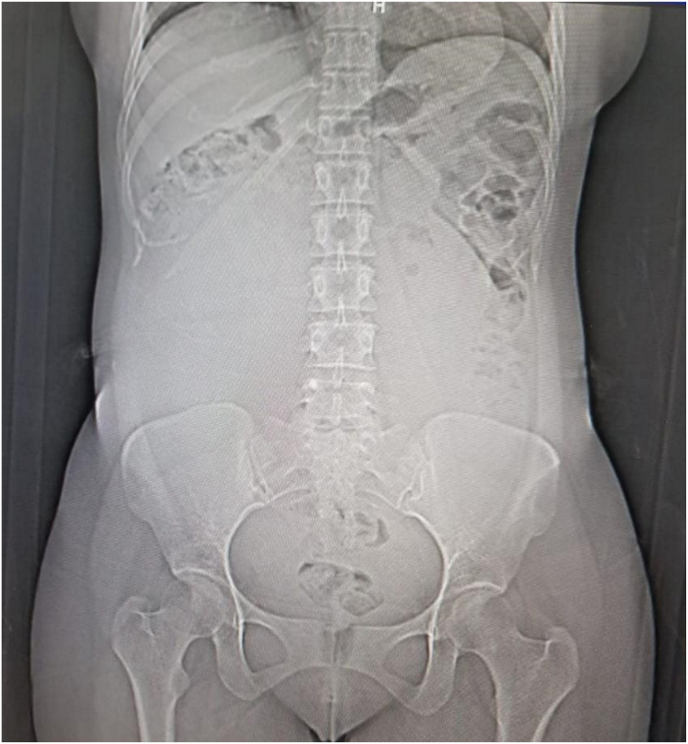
Abdominal radiograph showing a large soft tissue density, which displaced the bowels superiorly, likely to have originated from the pelvis. There is no calcification within the density.
